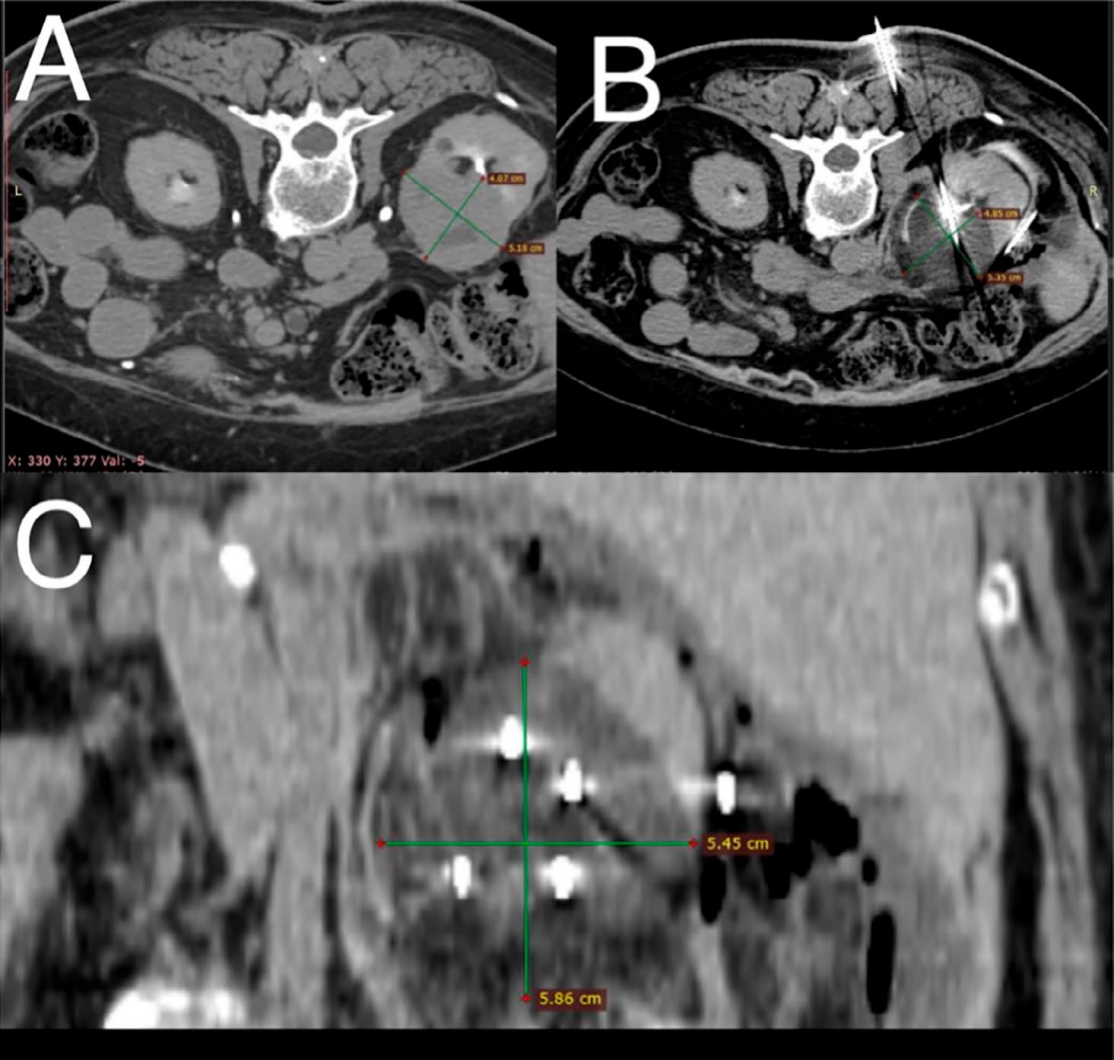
CT images before (A) and during (B) the cryoablation procedure. The hypodense iceball (C) created by multiple cryoprobes is completely covering the tumor, creating adequate margins. The intestine and the ureter were hydrodissected in order to perform a safe ablation.
